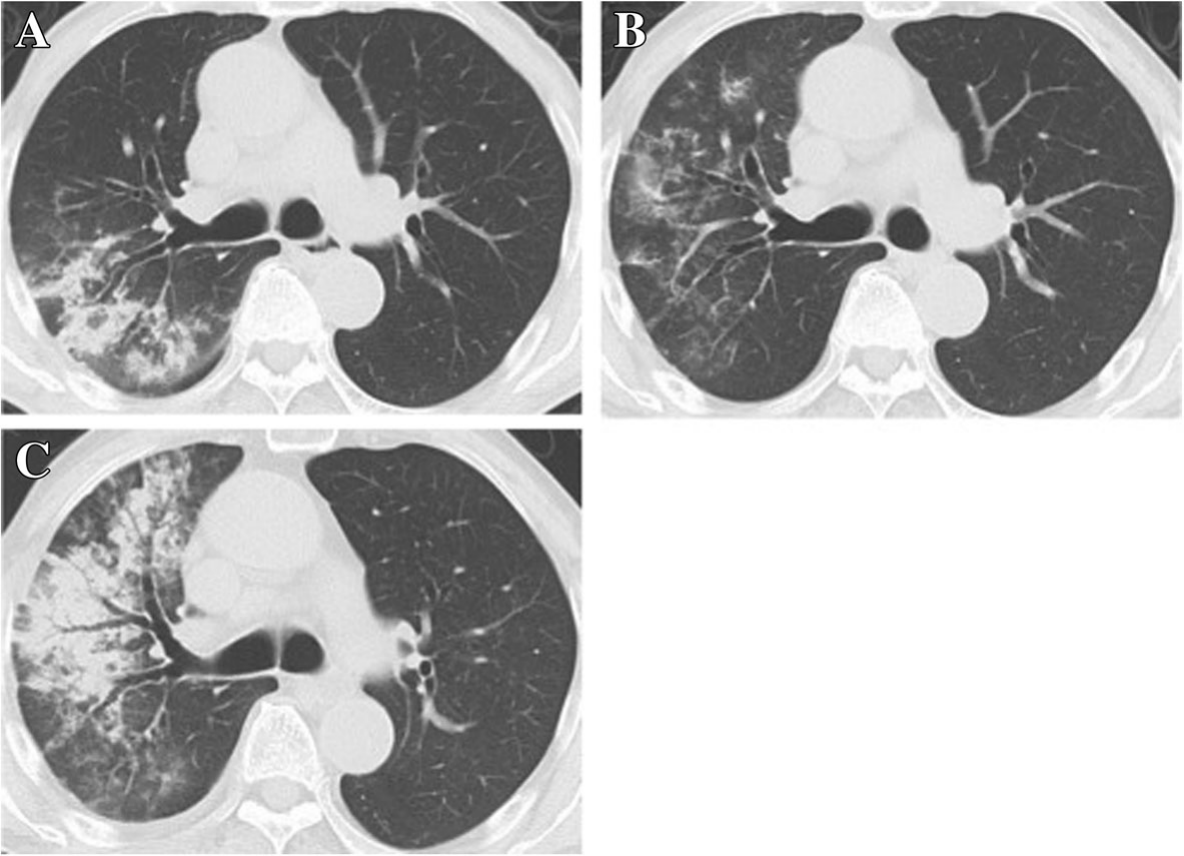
Typical CT images of relapse case. a Consolidation and nodules in the right upper lobe were recognized at the first visit. b These shadows remained without vanishing completely after treatment. c Relapse occurred after six months of treatment
